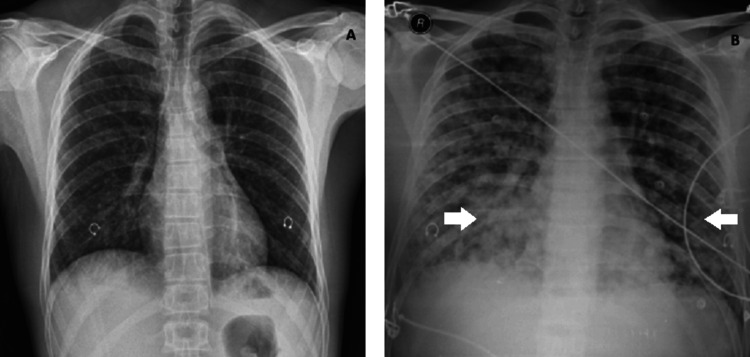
Evolution in chest radiograph at presentation, showing a normal image (A) and progression with bilateral diffuse pulmonary infiltrates (B)
